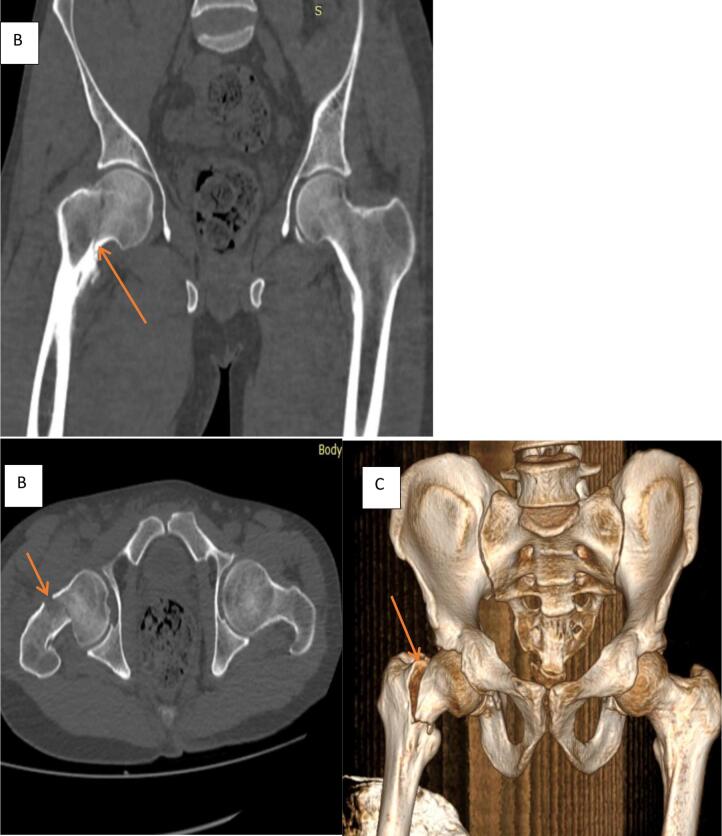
Pelvic CT Scan coronal and axial 3D rendering image demonstrating non-displaced trans cervical right femoral neck fracture orange arrows in A, B and C.
